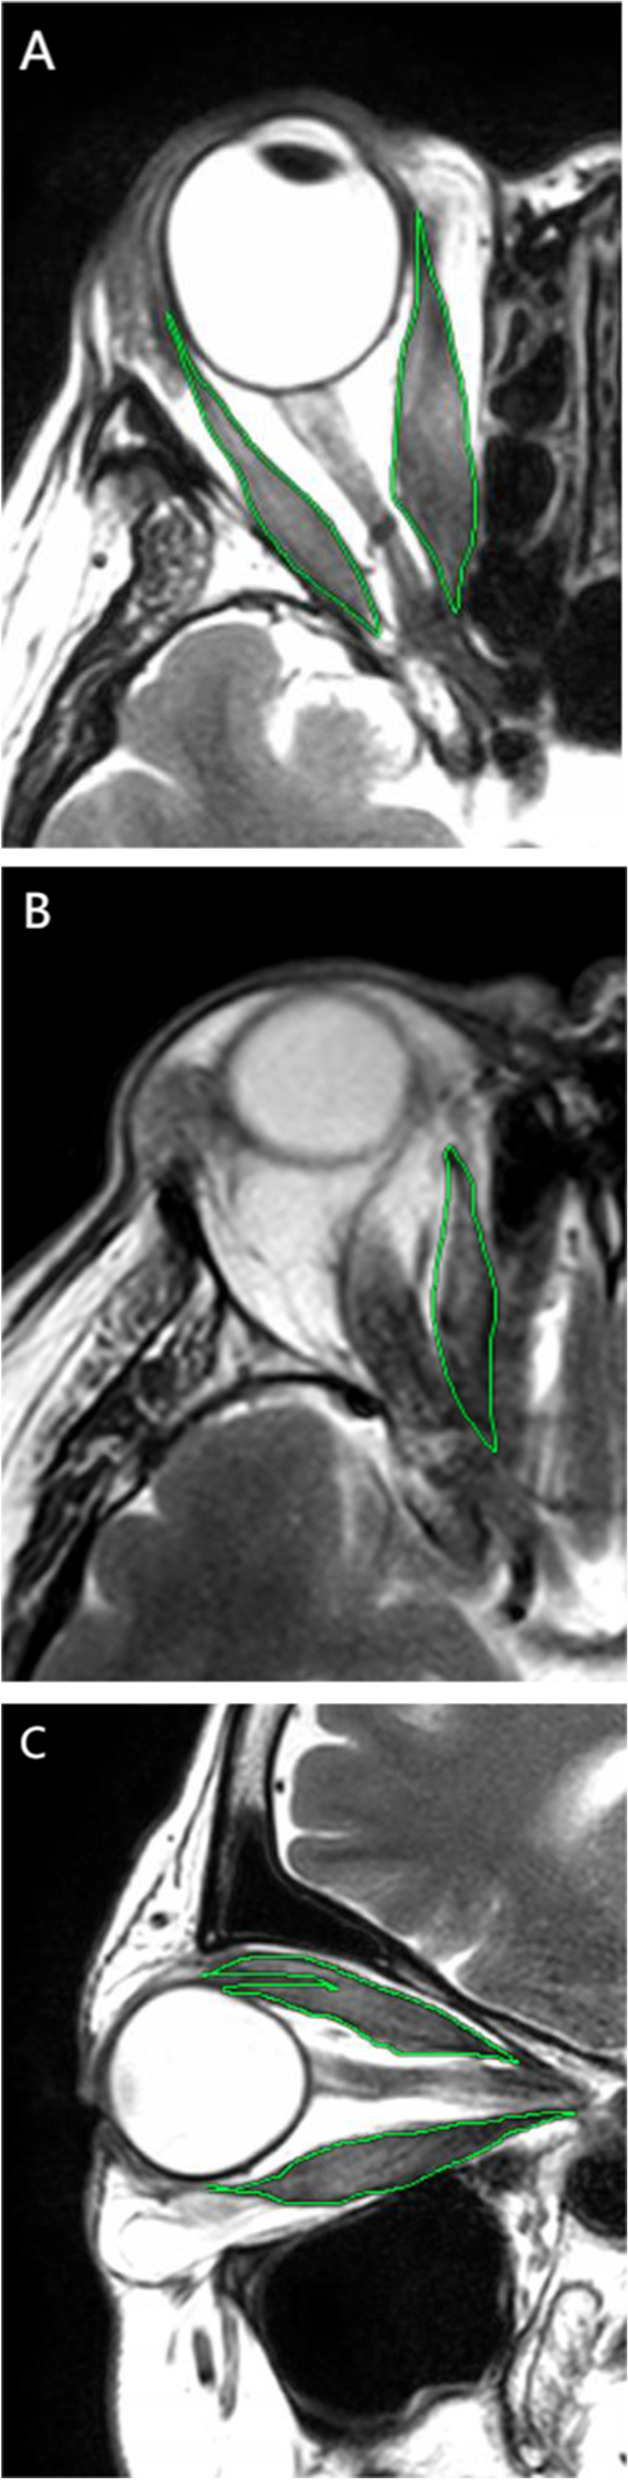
The cross-sectional area of the extraocular muscle was measured on T2-weighted magnetic resonance imaging scans. (A) The areas of the medial rectus and lateral rectus were obtained from axial images. (B) The areas of the superior oblique muscles were obtained from the axial images. (C) The areas of the superior rectus complex and inferior rectus were obtained from the sagittal images
